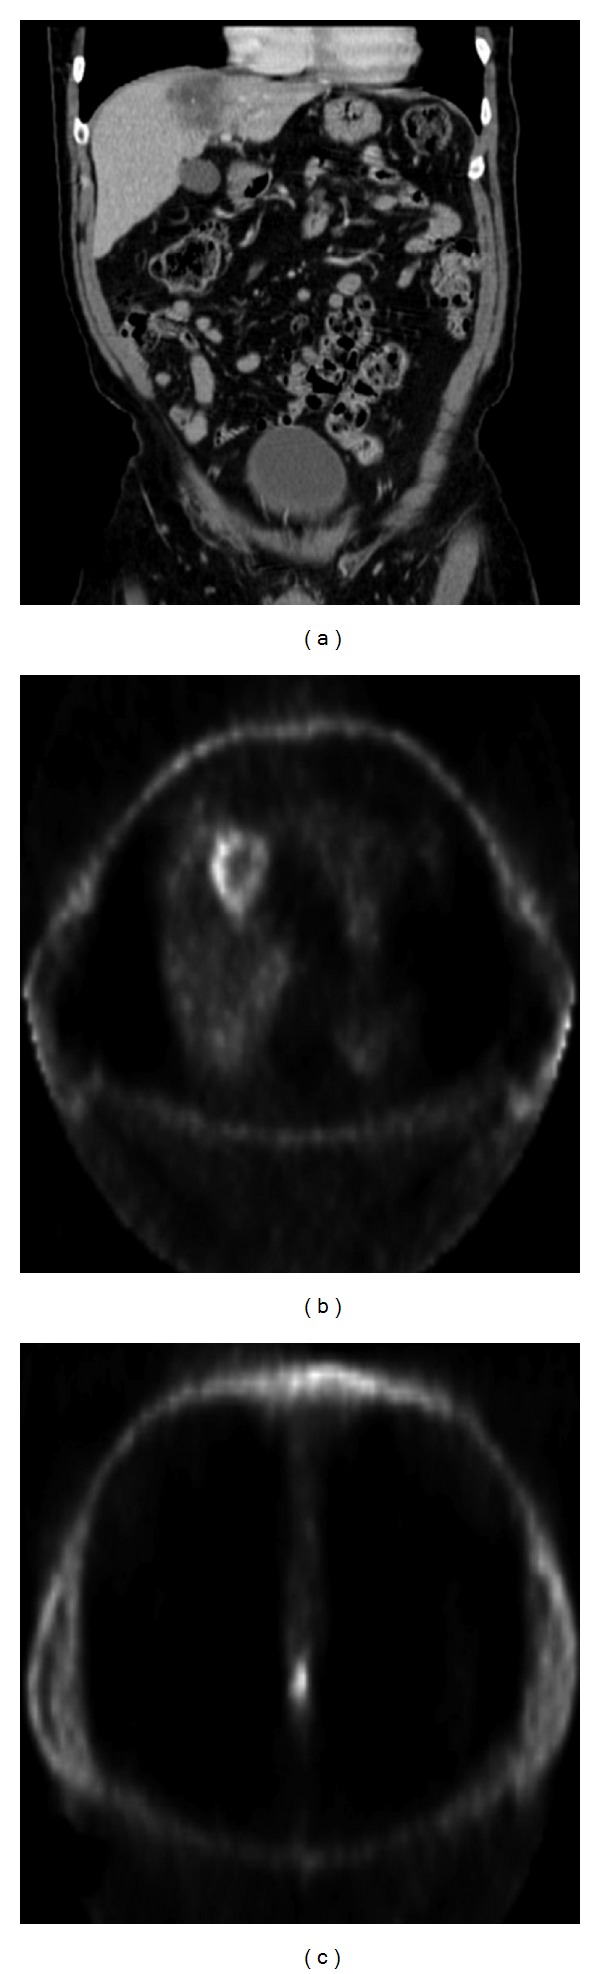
CT (a) and PET (b) showing hepatic metastasis, and (c) PET showing anal primary.
